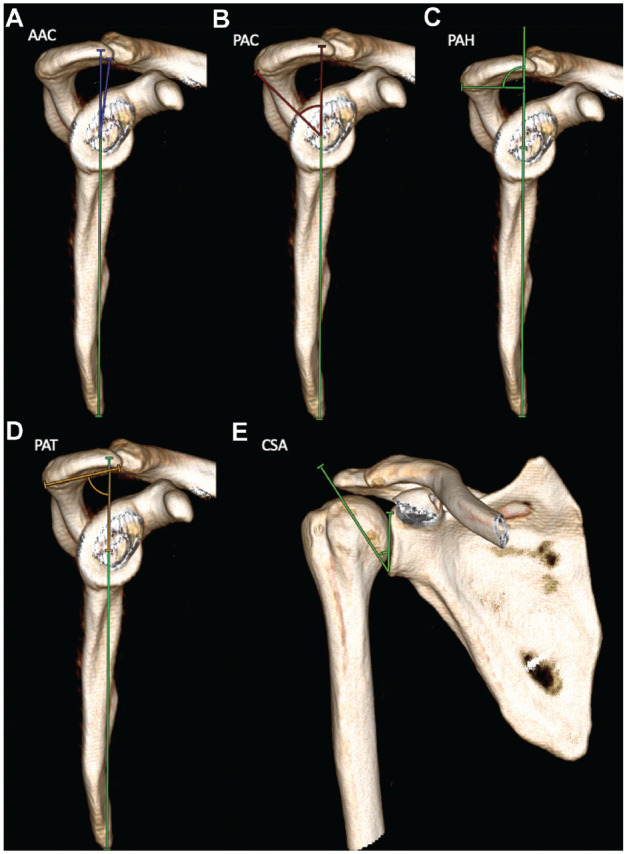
Measurements of the acromial morphology on 3-dimensional computed tomography models. (A) The AAC refers to an angle formed by the reference line connecting the inferior margin of the scapula with the center of the intersection of the small arms of the “Y” and a line drawn from the intersection of the small arms of the Y to the most anterior point of the inferior aspect of the acromion (blue angle). (B) The PAC refers to an angle formed by the same reference line and a line drawn from the intersection of the small arms of the Y to the most posterior point of the inferior aspect of the acromion (red angle). (C) The PAH is measured as the distance from the center of the intersection of the small arms of the Y to a perpendicular line that connects the reference line with the most posterior point of the inferior aspect of the acromion. (D) The PAT is measured as the angle formed by the reference line and a line connecting the most posterior point of the inferior aspect of the acromion to the most anterior point of the inferior aspect (brown angle).^
